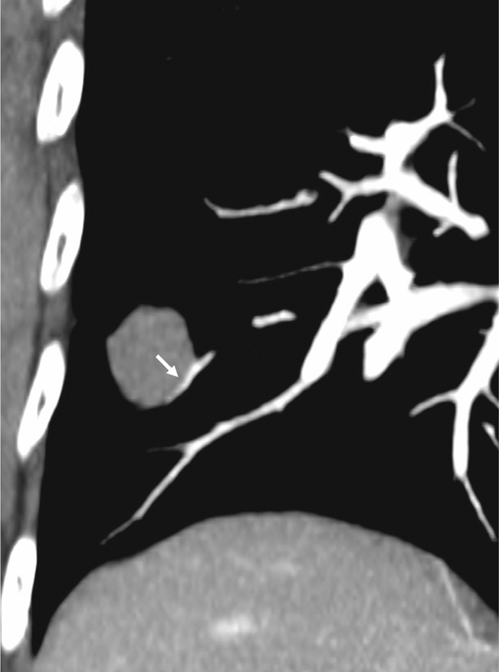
Displacement. This is a 68-year-old female with a pulmonary nodule in the right lower lobe. She received right lower lobe lobectomy, and pathology revealed adenocarcinoma. Coronal reformatted CT image reveals the nodule pushing the peripheral right pulmonary artery away from its normal vascular course with a smooth indentation on the pulmonary artery (white arrow).
